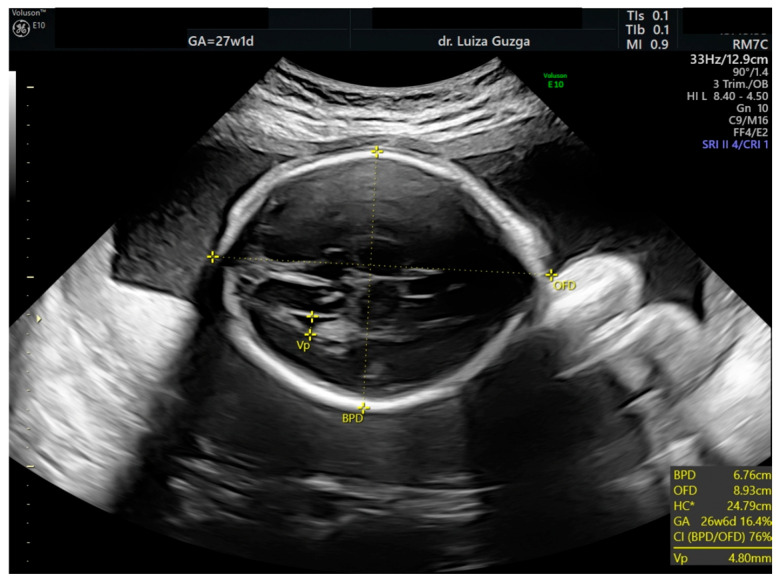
Two-dimensional ultrasound examination of the fetus at 27 weeks of gestation, revealing the abnormal shape of the head (trigonocephaly). Abbreviations: BPD (Biparietal diameter); HC (Head circumference); GA (Gestational age); Vp (Posterior ventricle); w (weeks); d (days).
